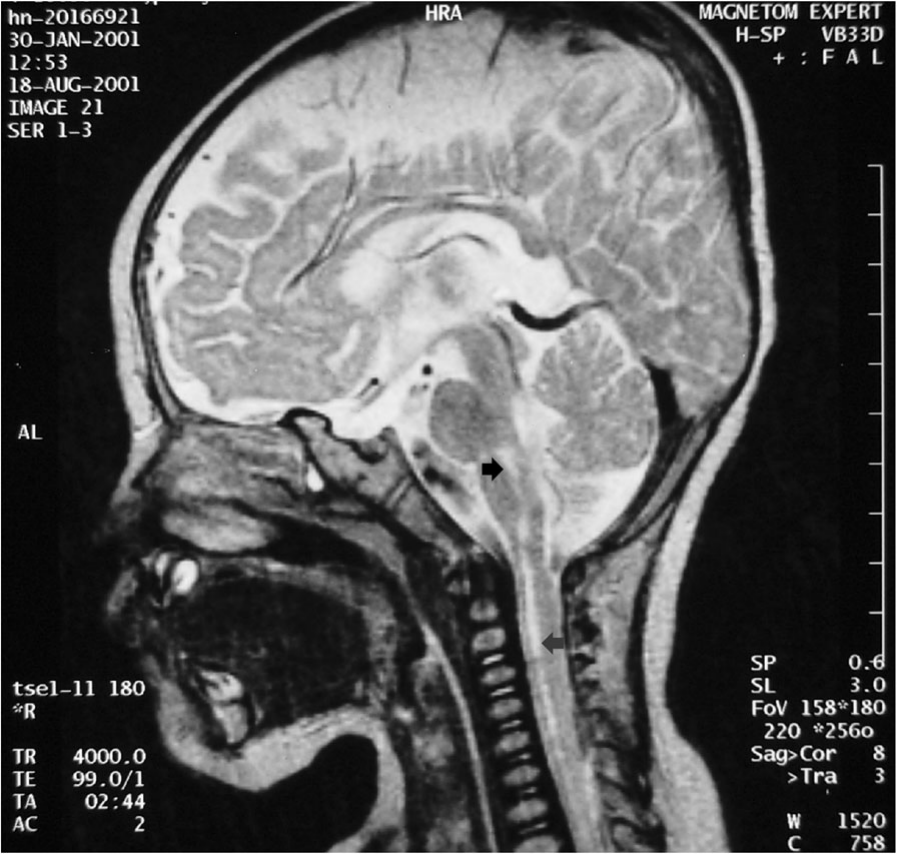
High-intensity lesions in the tegmentum of the brain stem (black arrow) and high-intensity lesions in the cervical spinal cord (gray arrow) on the T_2_-weighted image of MRI in a case with the sequelae of central hypoventilation and right upper limb weakness and atrophy
